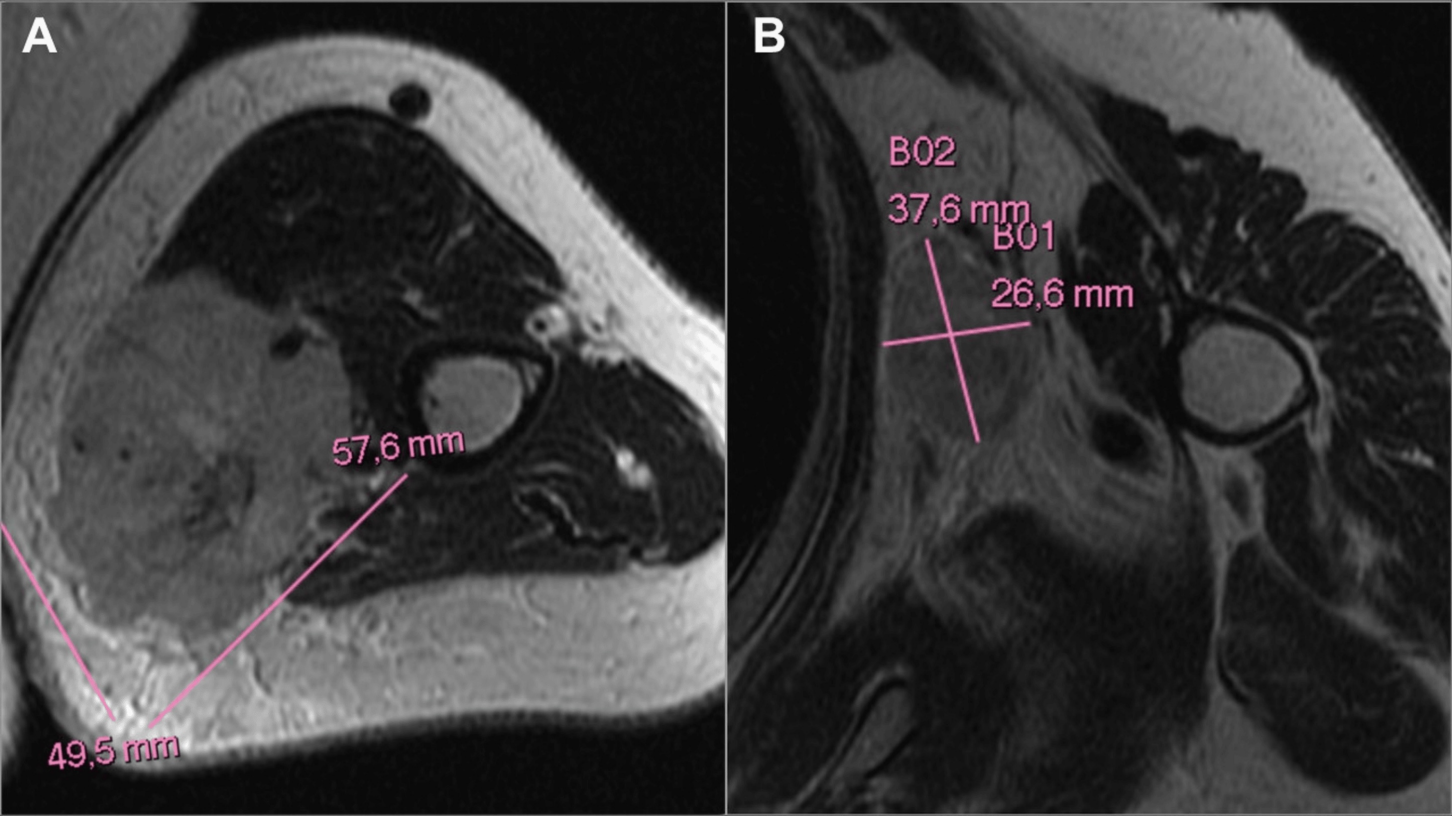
Initial magnetic resonance imaging MRI images show the left arm tumor and its relationship with the vasculonervous structures of the humeral bundle and axillary lymphadenopathy, respectively. (A) T2 sequence. (B) T1 sequence.
